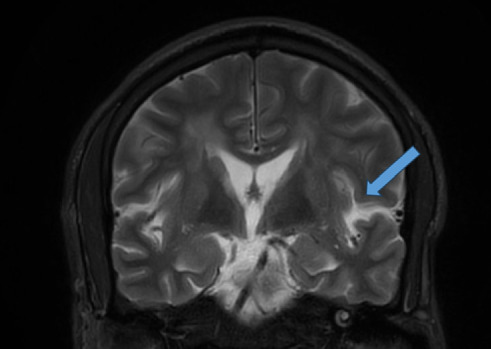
Head MRI. Coronal T2-weighted image demonstrates a focus of increased signal intensity in the right frontal operculum (blue arrow). Axial FLAIR image demonstrates extensive patches of increased signal intensity in the bilateral parieto-occipital subcortical white matter. Axial diffusion-weighted imaging (DWI) shows no restricted diffusion in the corresponding areas, consistent with vasogenic edema.
